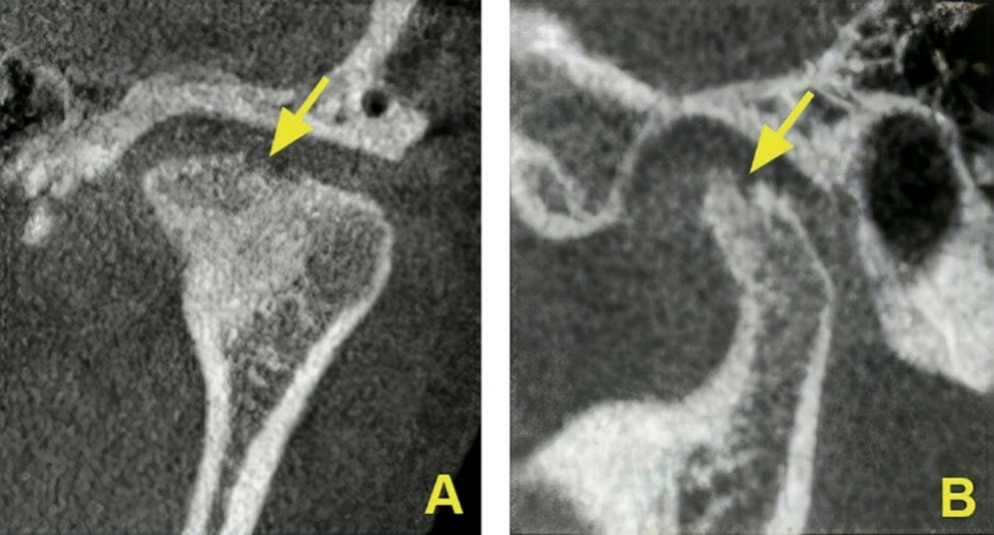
CBCT sections displaying condylar erosion. (A) Coronal plane. (B) Sagittal plane
